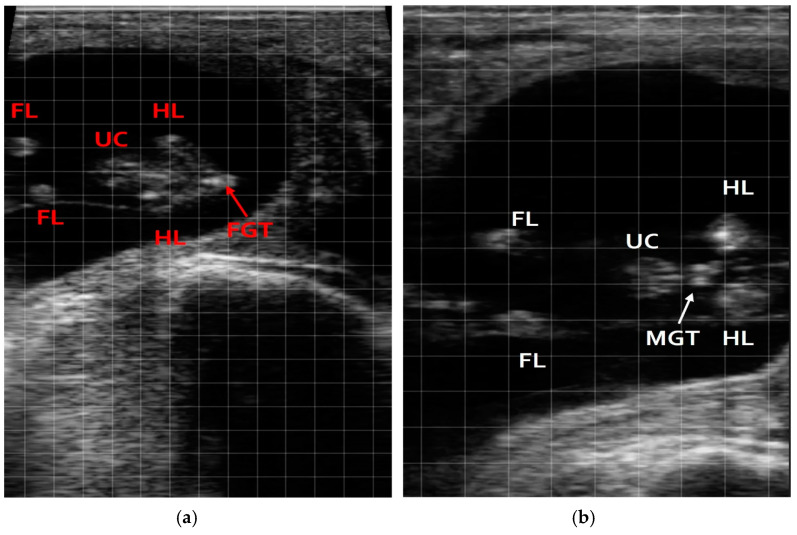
Still image ultrasound of a fetus between 50 and 70 days of pregnancy. (a) Female; (b) male, hind limbs (HLs); fore limbs (FLs); umbilical cord (UC); male genital tubercle (MGT); female genital tubercle (FGT).
